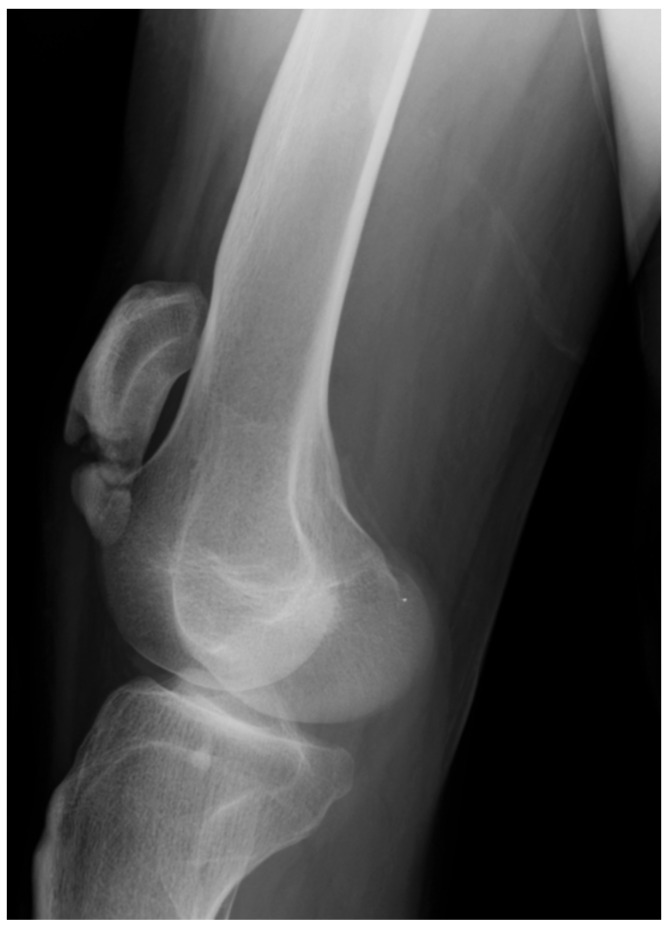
Radiographic features of Stage 4 MSP in the knee, in a 16-year old boy with severe crouch gait. There is marked patella alta and stress fracture of the patella with signs of healing with separation at the fracture site.
